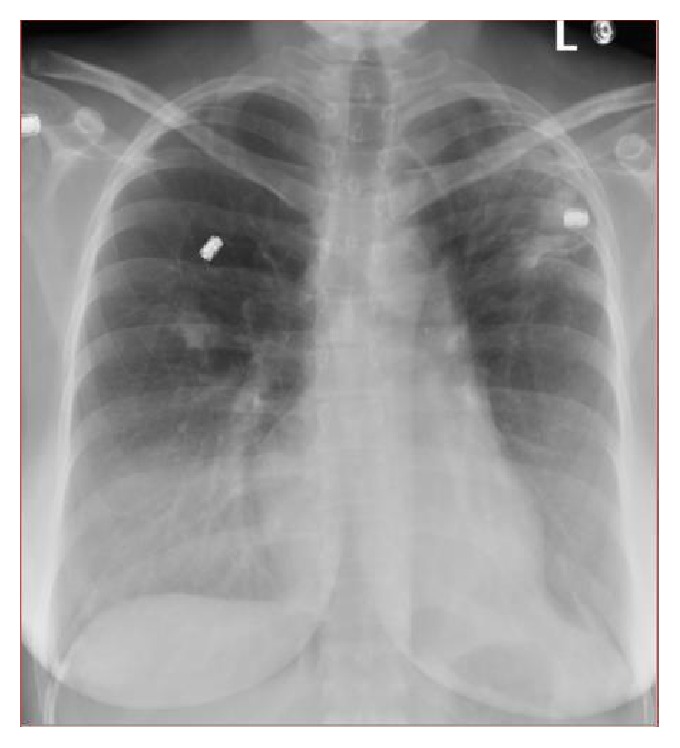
Chest X-ray. Increased density over the left upper lung and right middle lobe suspicious for infiltrate/fibrotic change.
